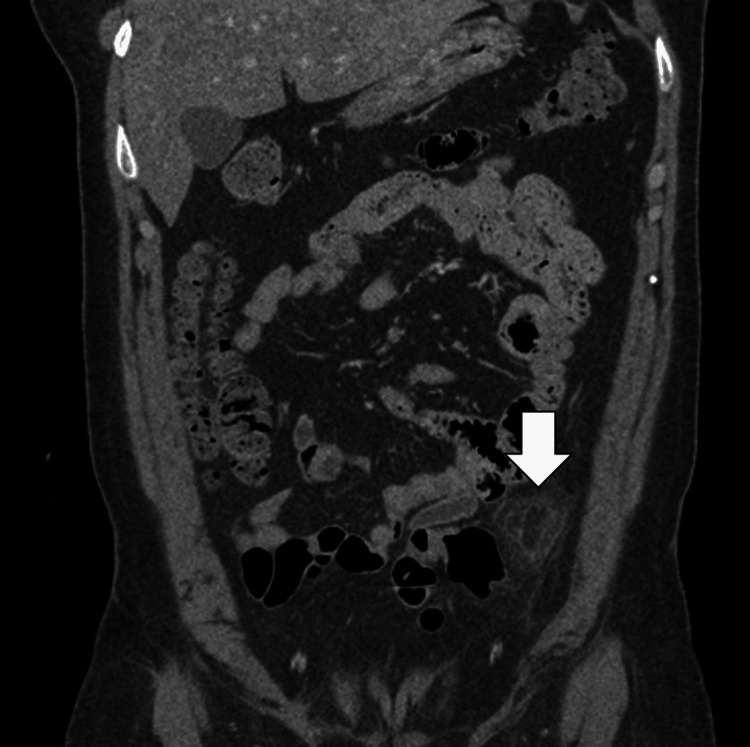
Coronal reformatted CT image showing an oval, fat-density lesion (arrow) along the serosal surface of the descending colon, consistent with acute epiploic appendagitis. There is no evidence of associated bowel wall thickening.
